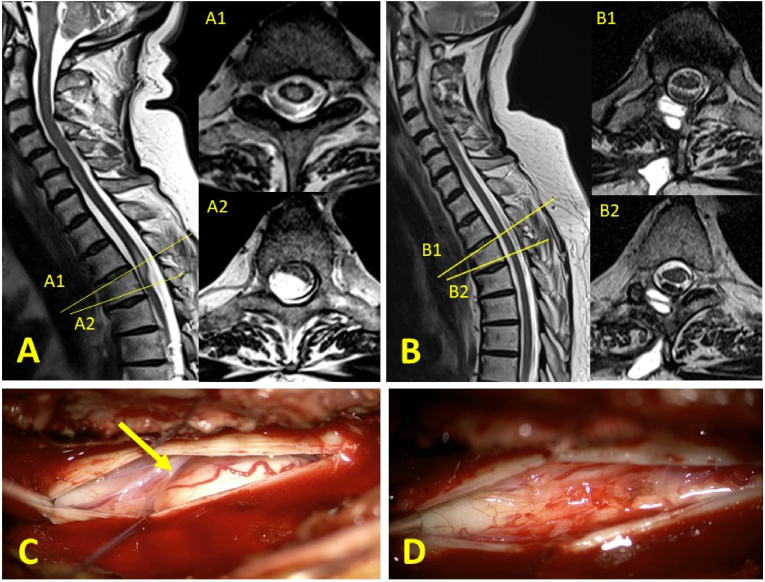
A: preoperative MRI of a patient with classical ‘scalpel sign’ on high-resolution T2-weighted MR imaging of the spinal cord. A1: axial plane at the segment of spinal cord lesion, A2: at the segment of the AW with maximal ventral myelon displacement/dorsal indentation. B: postoperative MRI of the same patient showing resolution of ventral myelon displacement and myelopathy, corresponding to postoperative clinical recovery. C: intraoperative view on the AW. D: after web removal.
